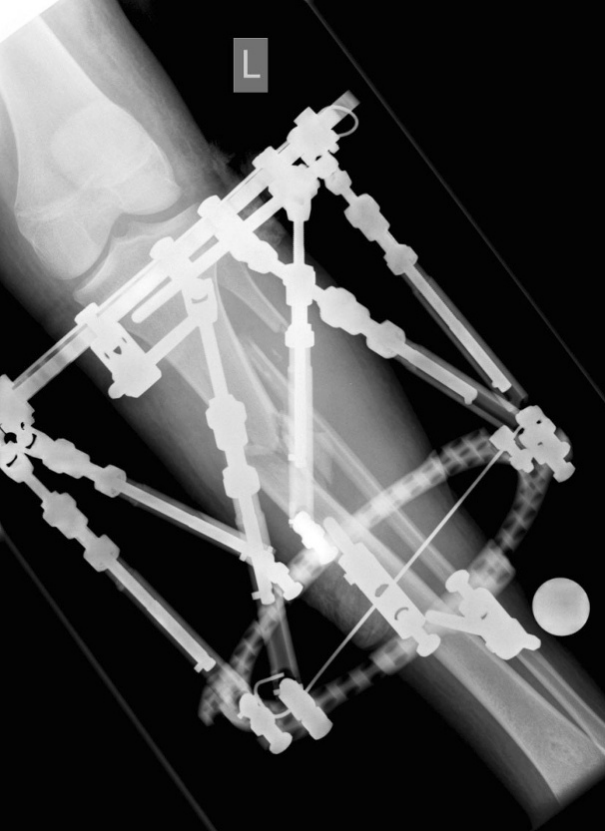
Immediate postoperative radiograph following stabilisation with TSF
